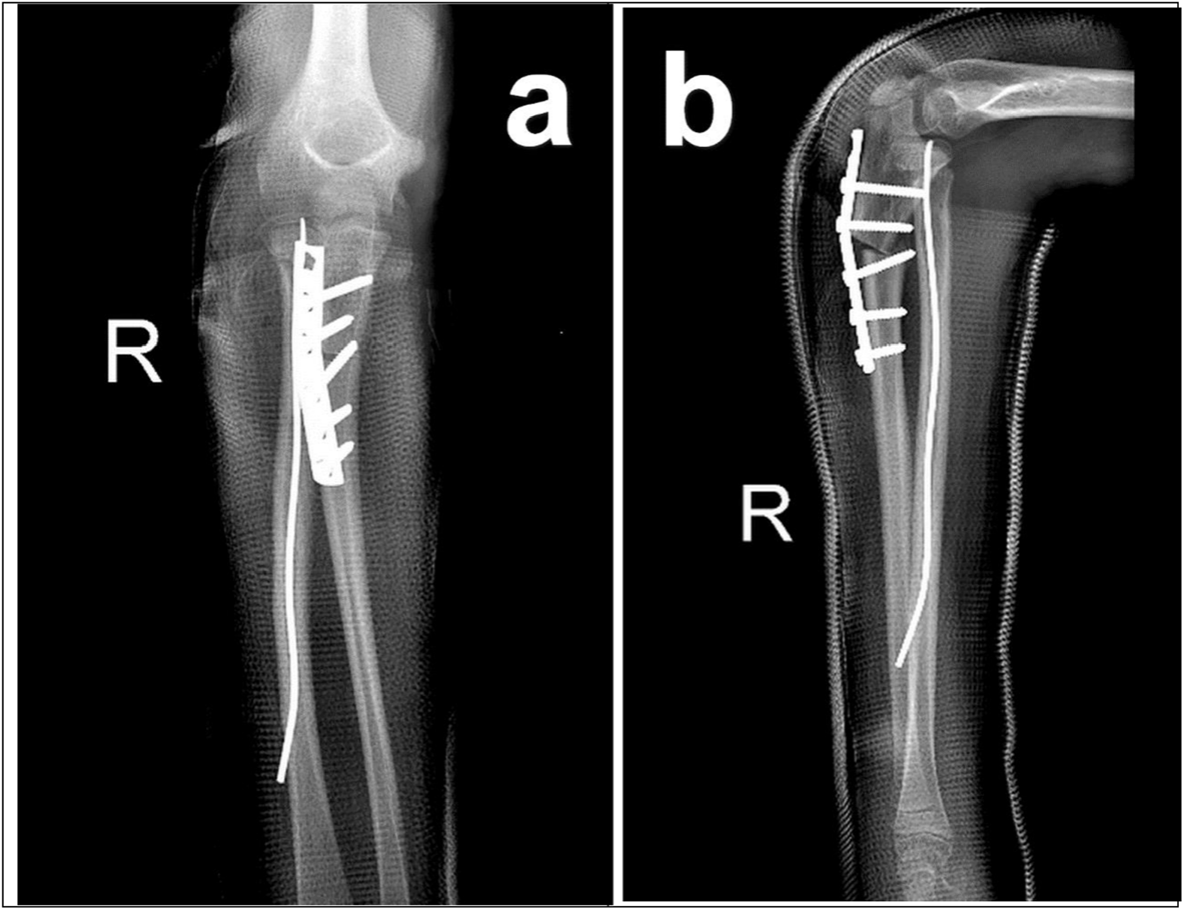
a Post-operational AP X-rays showing fixation of radial neck and proximal ulna with elastic intramedullary nail and plate/ screws. b Lateral X-rays showing fixation of radial neck and proximal ulna with elastic intramedullary nail and plate/ screws
